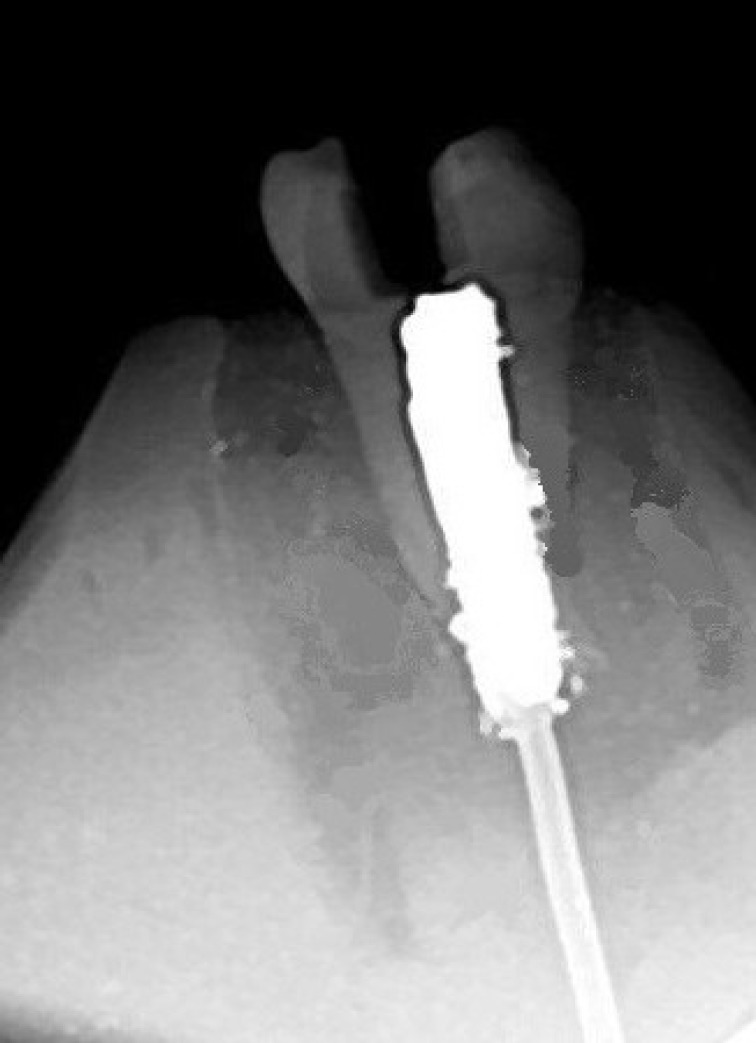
Radiographic confirmation of thermocouple in the pulp chamber
